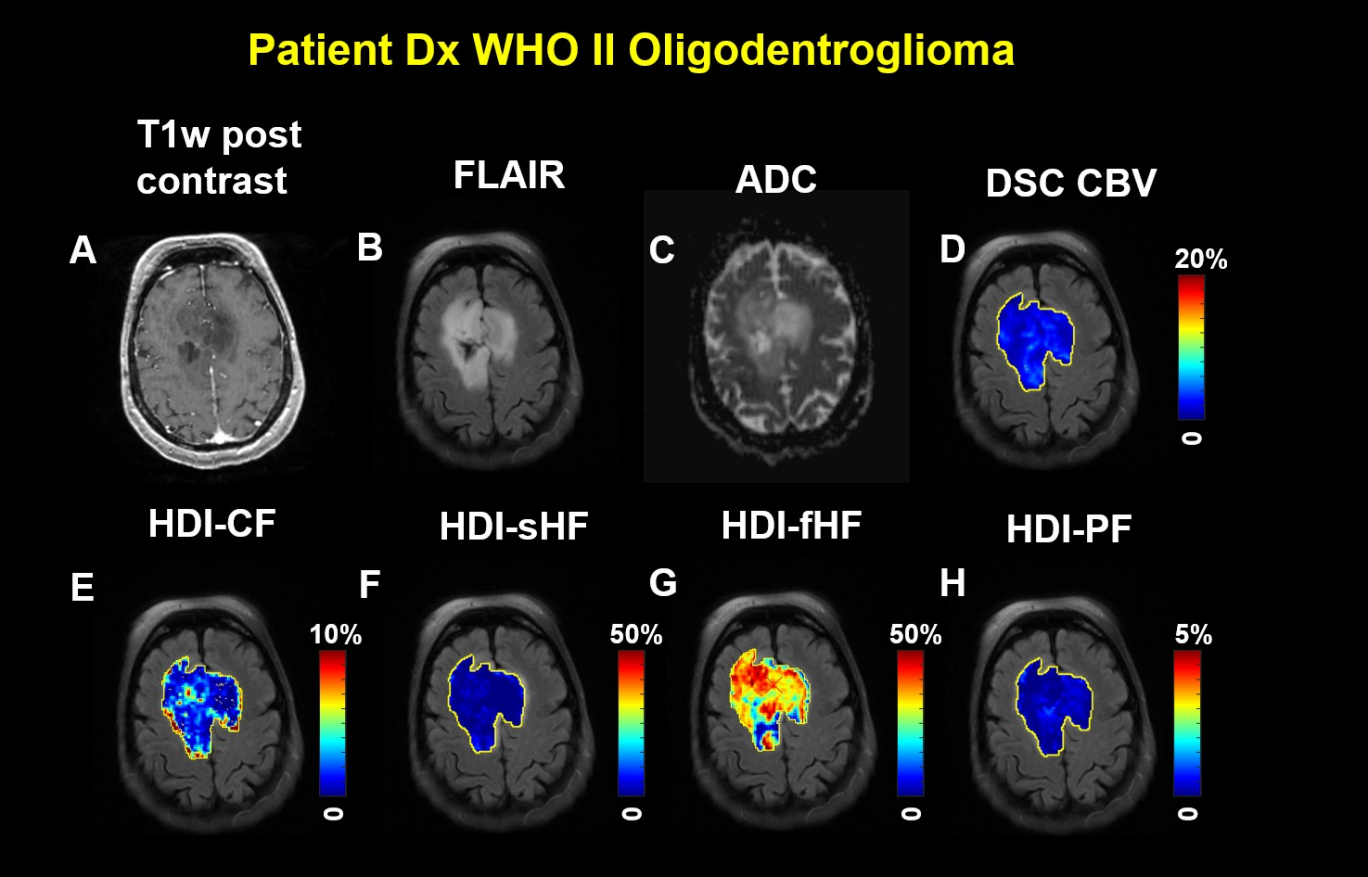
Imaging from a woman in her 70s diagnosed with World Health Organization grade II recurrent oligodendroglioma. (A) The T1-weighted post-contrast image shows a lesion with decreased signal intensity. (B) The fluid-attenuated inversion recovery image and (C) the diffusion magnetic resonance imaging-derived apparent diffusion coefficient show a lesion with an increased signal. (D) The dynamic susceptibility contrast-derived cerebral blood volume map and the Heterogeneity Diffusion Imaging-derived (E) cellularity fraction, (F) slow hindered diffusion fraction, (G) fast hindered diffusion fraction, and (H) perfusion fraction maps were generated on manually defined tumor regions and overlaid on the fluid-attenuated inversion recovery image. No elevated cerebral blood volume and Heterogeneity Diffusion Imaging-derived slow hindered diffusion fraction and perfusion fraction are shown in the tumor region. The elevated Heterogeneity Diffusion Imaging-derived cellularity fraction and fast hindered diffusion fraction are shown in the tumor region.
